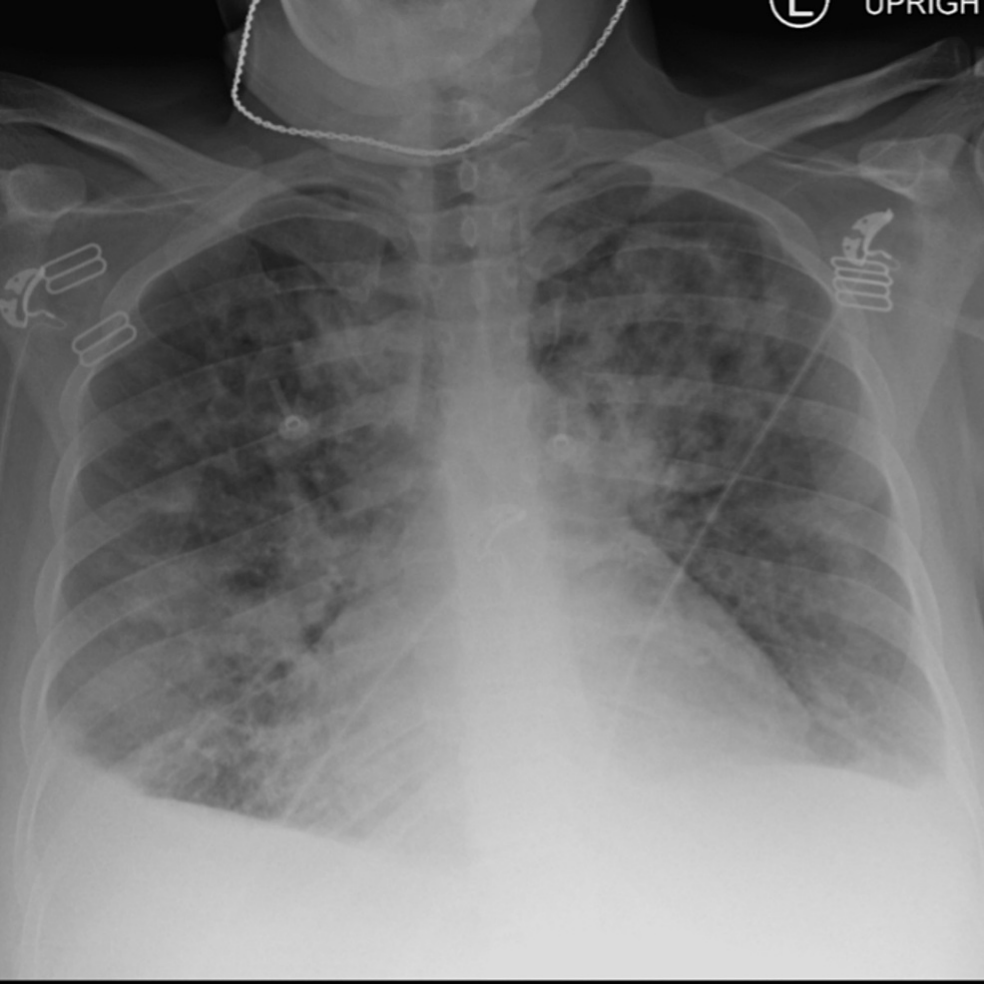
Chest X-ray Diffuse pulmonary vascular congestion was present with no evidence of pneumothorax.
